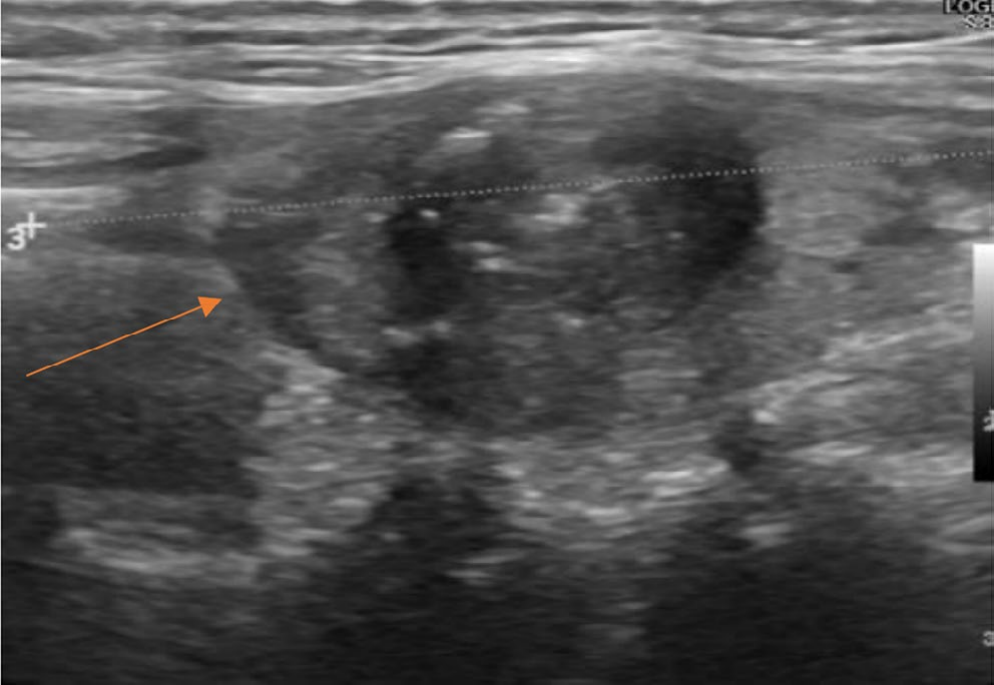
Thyroid ultrasound. A thyroid nodule measuring 14 × 20 × 20 mm (ACR‐TIRADS 5), is identified. The lesion is highly suspicious for malignancy, with potential capsular invasion.
